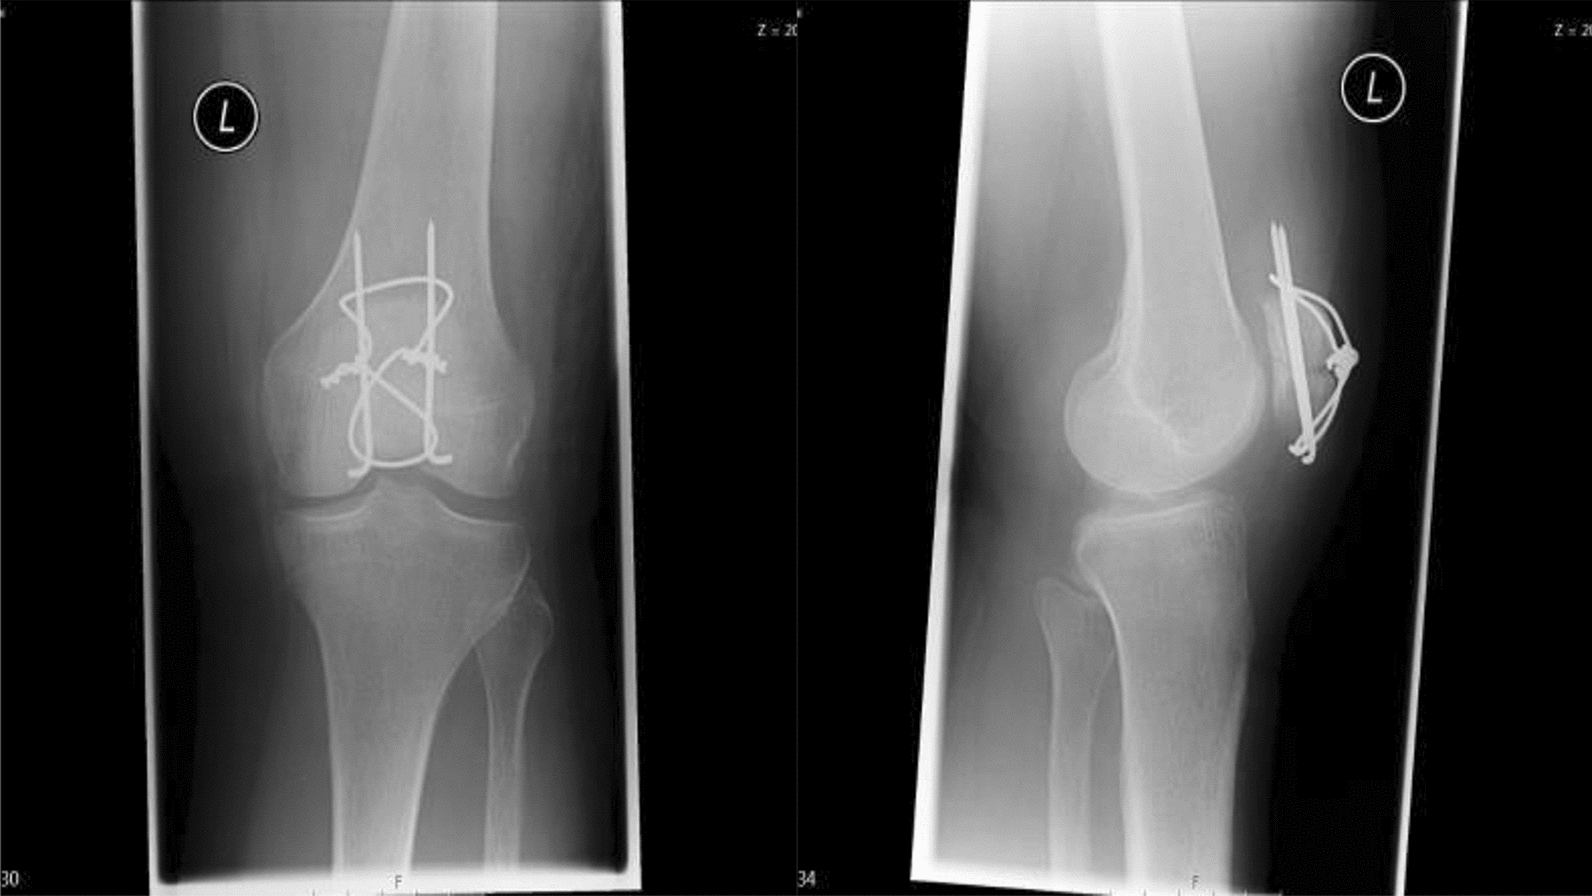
a/p (c) and lateral (d) X-ray of a transverse patella fracture after operative treatment using tension-band wiring
